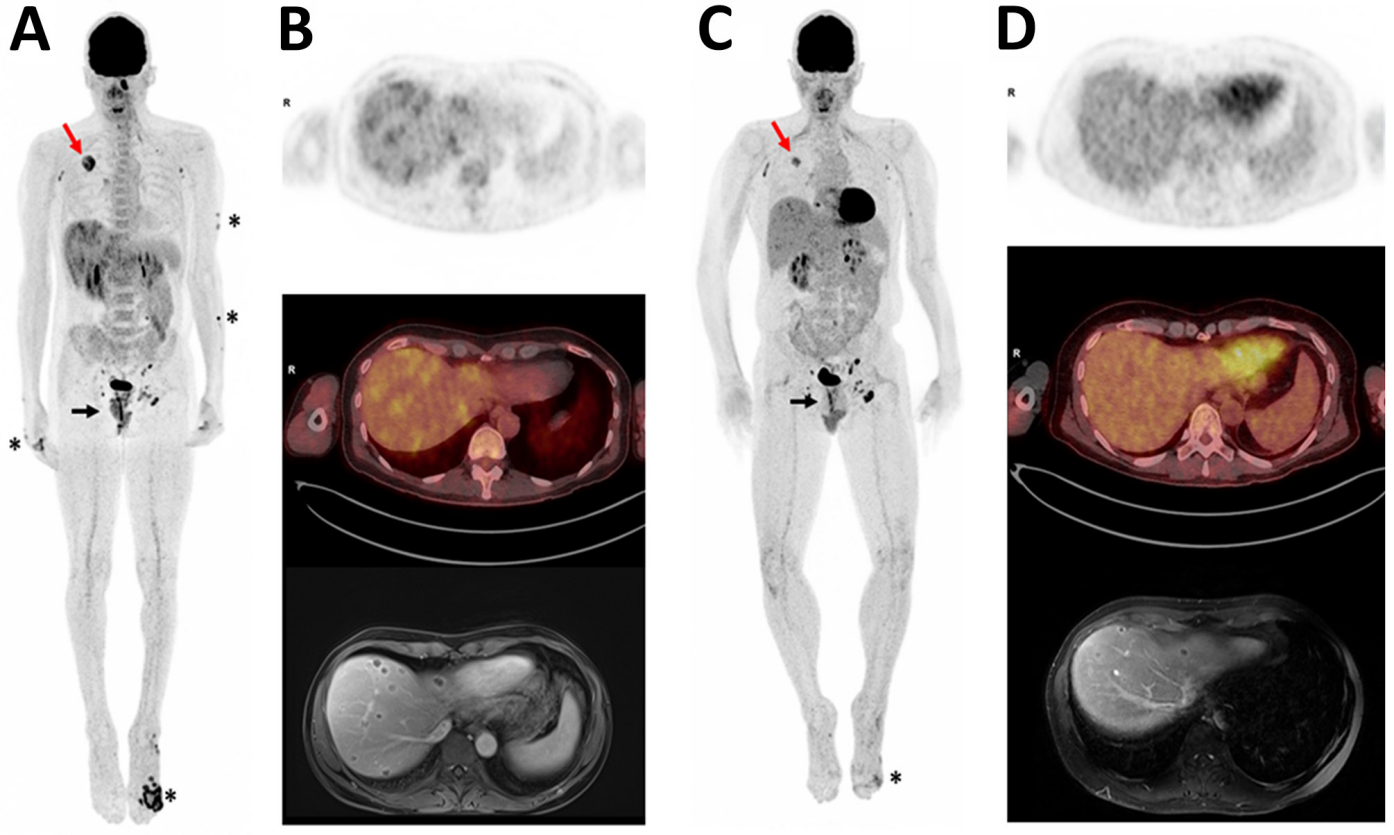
Positron emission tomography/computed tomography (PET/CT) scans and magnetic resonance imaging (MRI) of HIV/hepatitis B virus co-infected patient in case study of mpox hepatic and pulmonary lesions, France. A) Whole-body ^18^F-fluorodeoxyglucose (^18^F-FDG) PET/CT 3-dimensional maximum intensity projection performed in December 2022. Asterisks indicate anterior view of skin lesions. Black arrow indicates lesions in lower rectum; maximum standard uptake value (SUVmax) = 7. Red arrow indicates tumor in upper right lung; SUVmax = 6.4. Heterogeneous hepatic metabolism and multiple small foci of uptake were observed on liver transaxial views, which showed more intense metabolic ranges in the subcapsular region (SUVmax = 3.6). A hypermetabolic contralateral apical pulmonary nodule (SUVmax = 2.7) was also observed but is not visible in this image. B) Upper image shows liver transaxial ^18^F-FDG PET, middle image is fused PET/CT, and lower image is liver MRI (axial liver acceleration volume acquisition). C) Follow-up whole-body ^18^F-FDG PET/CT 3-dimensional maximum intensity projection performed in January 2023. Anterior view indicates substantial decreases in metabolic uptake intensities in foot lesion (asterisk), rectum (black arrow), and right lung tumor (red arrow). Tumor was 36 mm versus 46 mm in December, SUVmax 3.9 versus 6.4. Decrease in left apical pulmonary nodule, 9 mm versus 11 mm, SUVmax 1.3 versus 2.7; nodule was not visible in this image. D) Follow-up images indicate substantial decrease of liver abscesses. Upper image shows liver transaxial ^18^F-FDG PET, middle image is fused PET/CT, and lower image is liver MRI (axial T1 fat suppression volumetric interpolated breath-hold examination portal).
